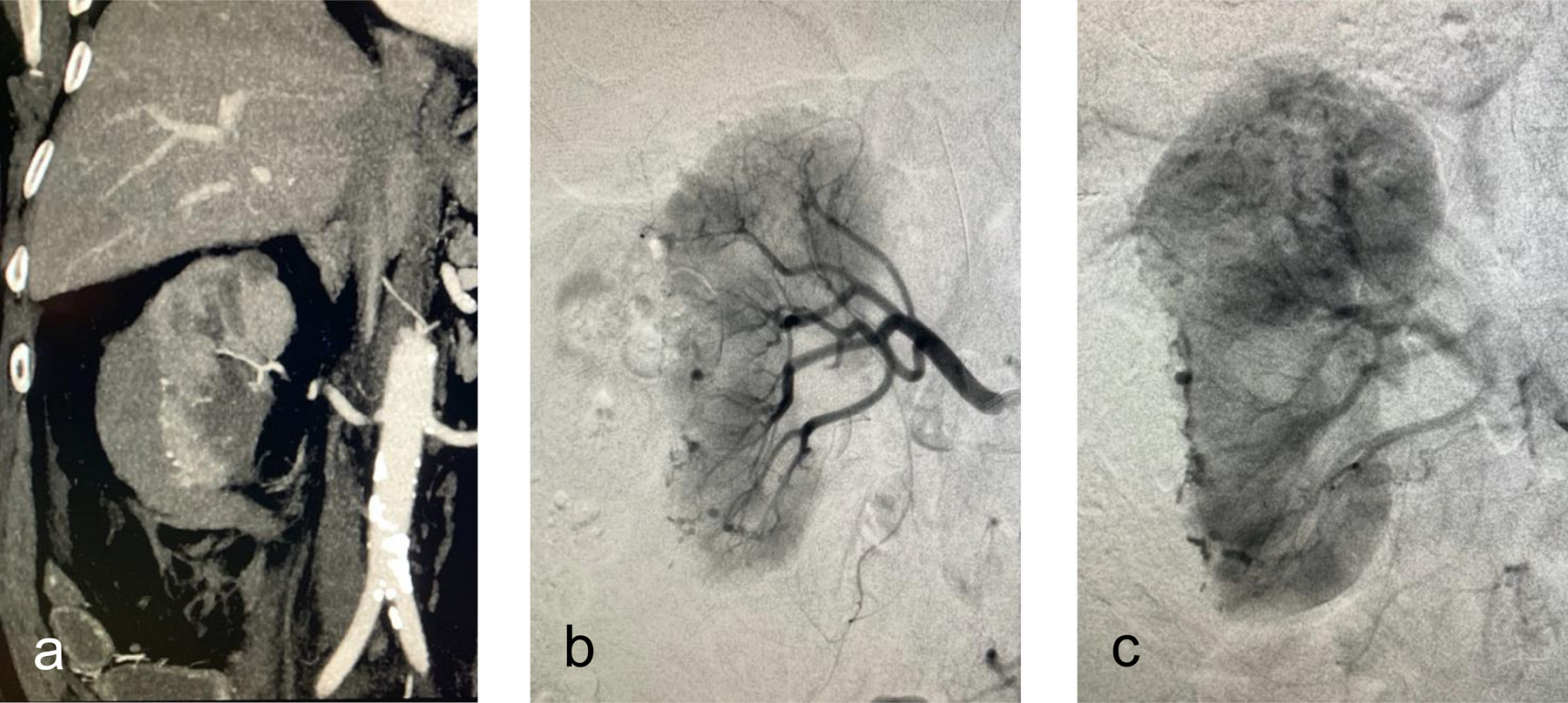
(A) Coronal CT with contrast on post-op day #1 shows a large subcapsular hematoma and renal lacerations associated with multiple pseudoaneurysms. (B) Early arterial phase selective right renal arteriogram demonstrates innumerable subcapsular pseudoaneurysms in the interpolar and lower pole regions, as well as suspicious areas of extravasation in the upper pole region (C) Late arterial phase selective right renal arteriogram confirms areas of extravasation peripherally throughout the entire kidney, some at a considerable distance from the ablation zone.
